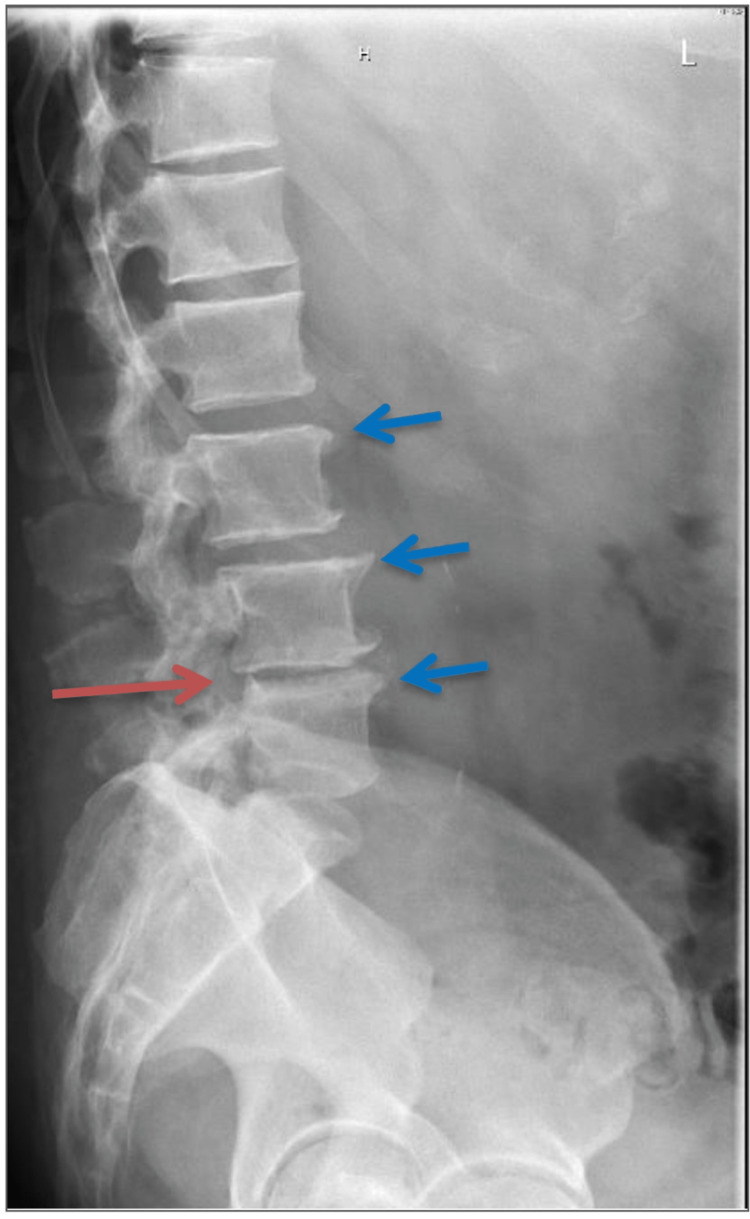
X-ray of the lumbar spine (lateral view) showing the anterior spurs in L3-L5 (blue arrows) and the posterolisthesis of L4-L5 (red arrow).
